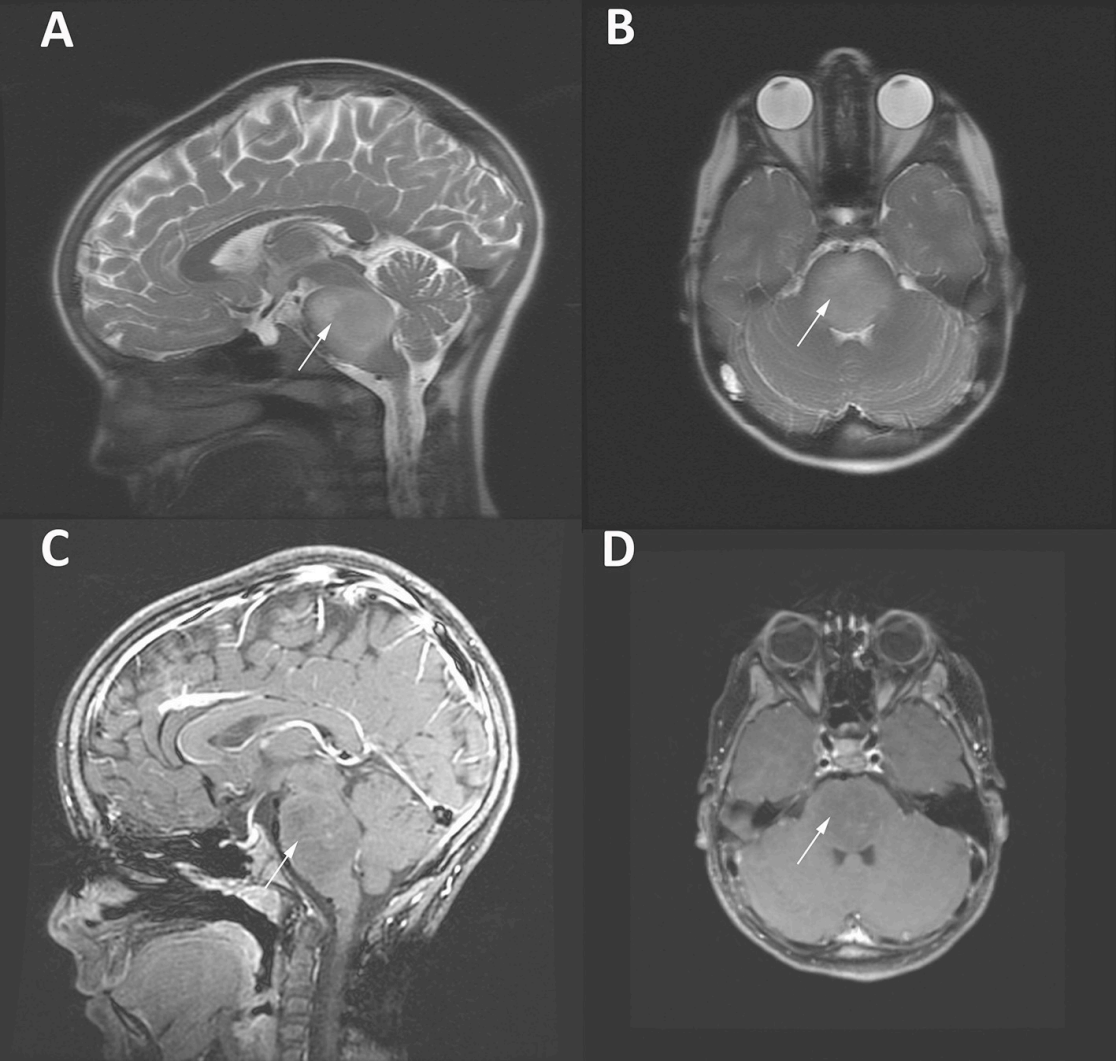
MRI imaging of an 8 year-old girl with a DIPG tumor (white arrows). T2-weighted sagittal (A) and axial (B) images demonstrate the enlargement of the brainstem and highlight the diffuse infiltrative characteristic of DIPG tumors. (C,D) Gadolinium-enhanced T1-weighted MRI images of the same patient demonstrating scant patchy enhancement.
